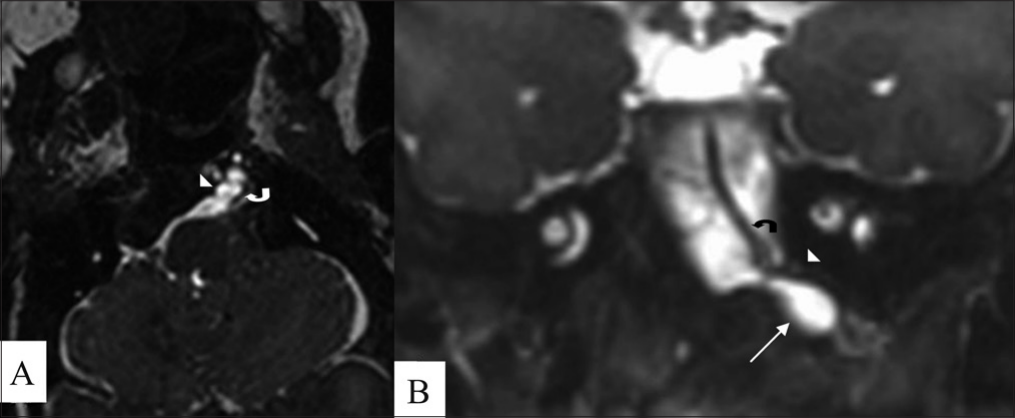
Axial (A) and coronal reformatted (B) constructive interference steady state (CISS) MRI images show herniation of a CSF sac (arrow) through a deficient left hypoglossal canal (arrowhead); there is also a vascular channel (curved arrow) passing through the canal
